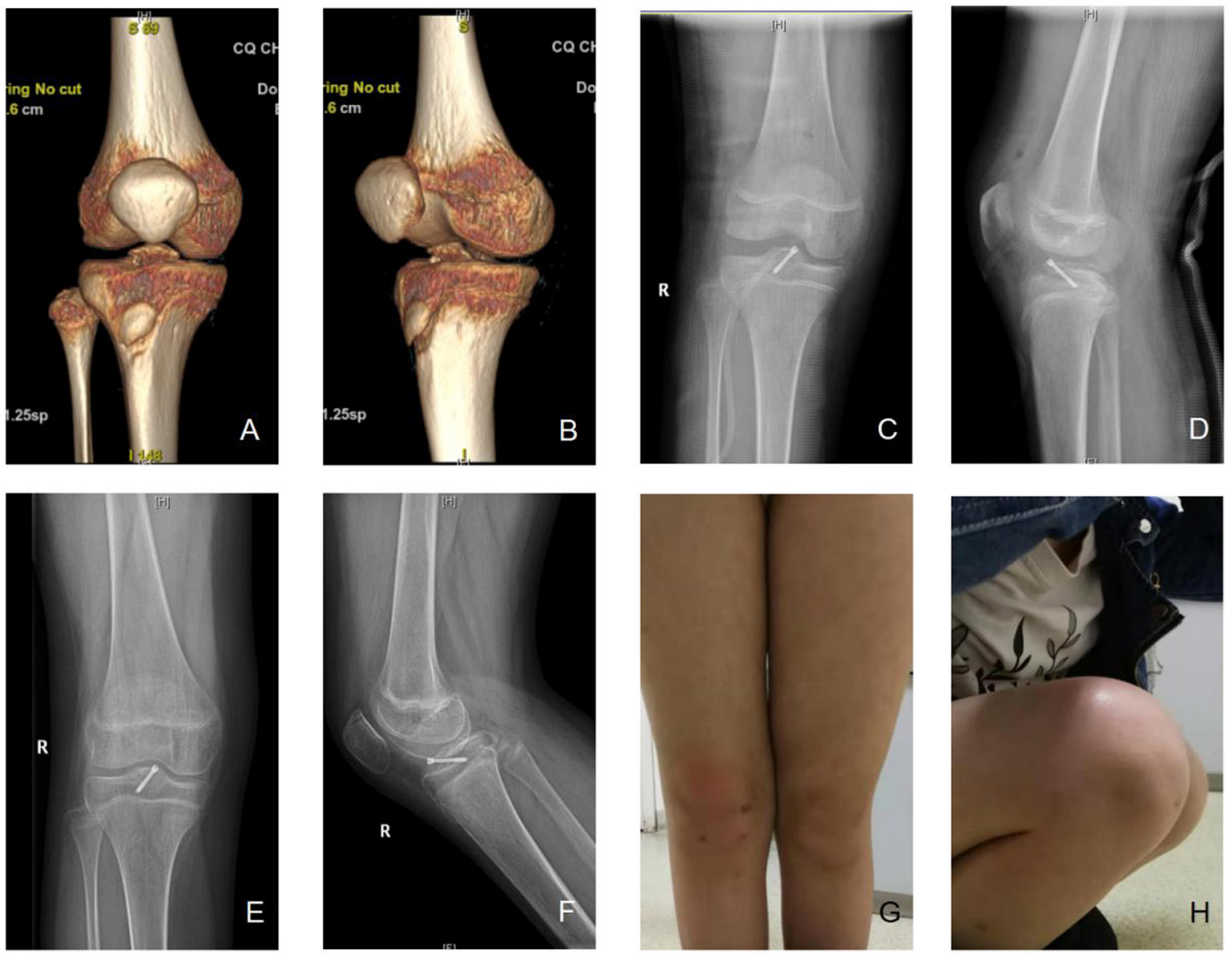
A typical case. (A,B) A 12-year-old female patient was diagnosed as tibial intercondylar eminence fractures, right, type III. Preoperative three-dimensional CT were shown. (C,D) Arthroscopic assisted internal fixation with hollow screws was applied. Postoperative X-ray showed good alignment of fracture. (E,F) 20 weeks after the operation, the X-ray showed that the fracture healed. (G,H) The appearance was good, and the flexion and extension activity of the knee was normal. The front drawer test, lackman test, and Pivot-shift test were negative, Lysholm score was 98, IKDC 96.6, Tegner score 8. No non-union, loosening of screw, or growth retardation of epiphyseal plate was observed.
